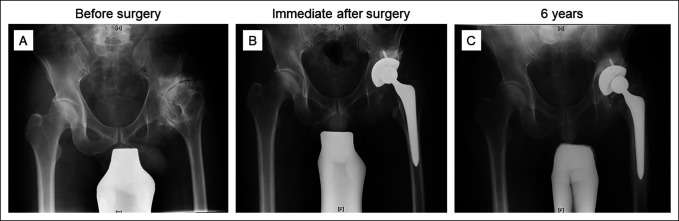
A, Radiographs showing a 58-year-old man presented with left hip pain owing to end-stage osteoarthritis secondary to dysplasia. B, Primary total hip arthroplasty using an Elance stem was done. C, The stem subsided by 26.4 mm at 6 years after surgery, which resulted in a shortened limb. He was scheduled for revision surgery, but the schedule is being readjusted owing to the COVID-19 pandemic. He is still on a waitlist.
